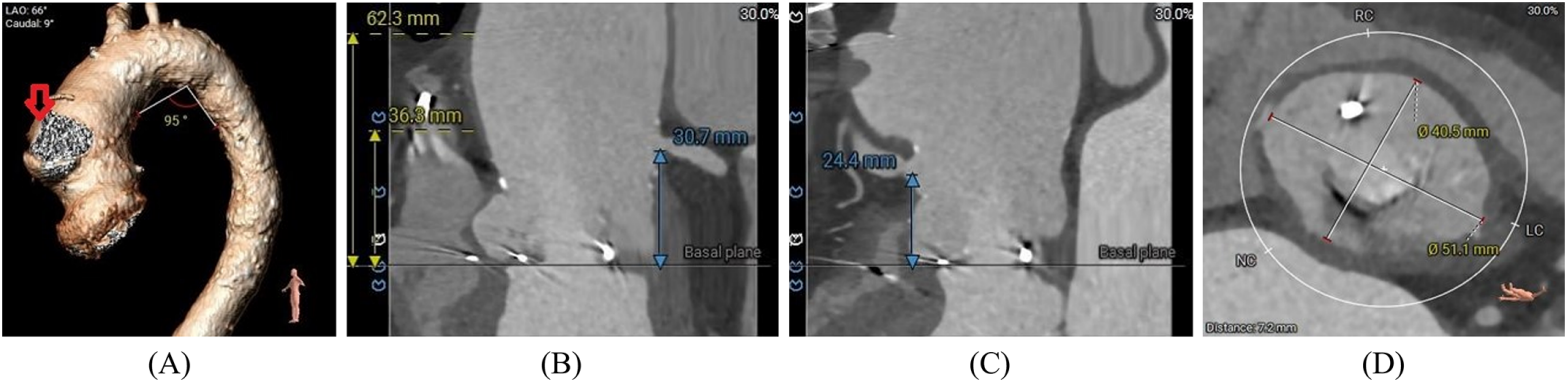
(A) Preoperative cardiac-gated CT images were utilized to create a 3D printed model with true-size aortic root dimensions, demonstrating a pseudoaneurysm (red arrow) of the ascending aorta. (B,C) The distance between the annulus and the ostium of the LCA and RCA. (D) The diameter of the sinus of Valsalva.
